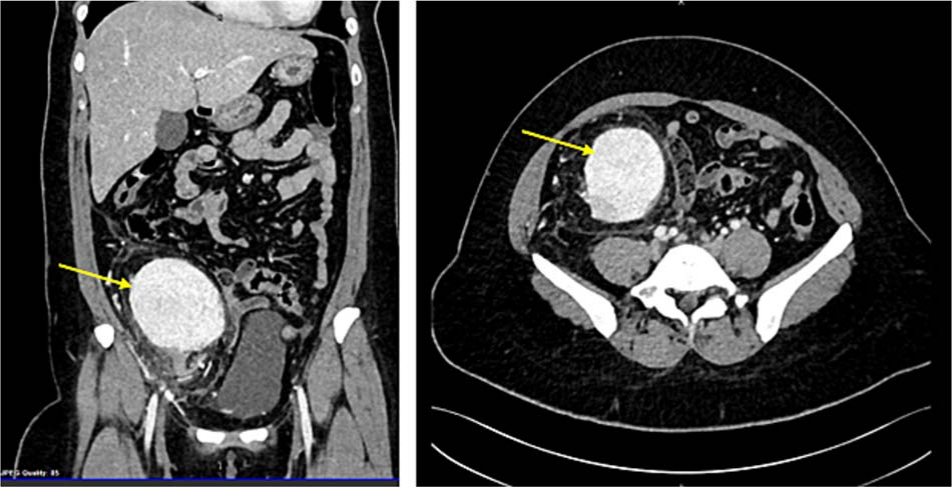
CT abdomen demonstrating the enlarged mass and its position by sagital and coronal sections (Arrow points at the mass).
